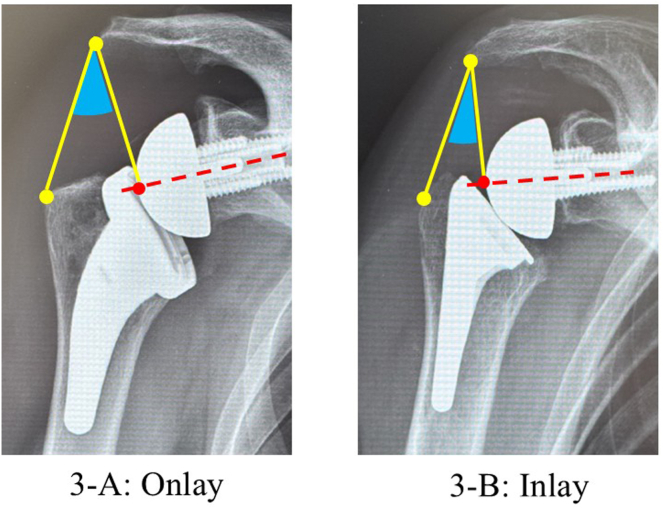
Comparison of HLA across implant types. Plain radiographs after rTSA are shown for both the onlay and inlay types. The humeral lateralization angle (HLA), indicated by the yellow line and blue-shaded area, is larger in the onlay-type. *rTSA*, reverse total shoulder arthroplasty.
